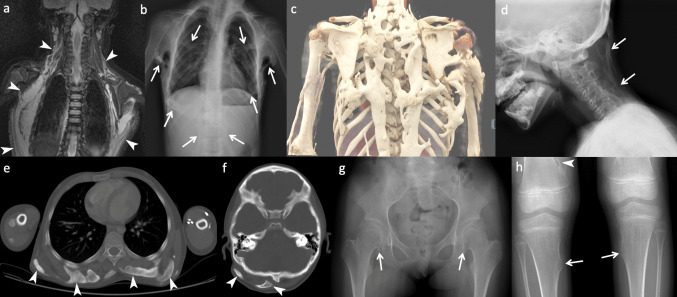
Imaging findings in fibrodysplasia ossificans progressiva (FOP). a Coronal short tau inversion recovery (STIR) image of a 13-month-old female (Patient 3) showing extensive myofascial edema (arrowheads) in the posterolateral thoracic wall at an early disease stage. b Follow-up radiography at age 6 years in the same patient demonstrates progression to heterotopic ossification of the thoracic wall, paraspinal, and axillary regions (arrows). c Three-dimensional volume-rendered image of an 8-year-old female (Patient 4) showing extensive, irregular ossifications involving the thoracic wall, paraspinal regions, and upper extremities. d Lateral cervical spine radiography of a 5-year-old male (Patient 8) demonstrating nuchal membrane ossification (arrows) and cervical facet joint ankylosis from C2 to C7, with enlarged posterior elements and hypoplastic vertebral bodies. e, f Axial CT images of the thorax (e) and head (f) from the same patient as in (d) showing thick subcutaneous, fascial, and/or intramuscular ossifications (arrowheads); the scalp lesion corresponds to a clinically described scalp nodule. g Anteroposterior (AP) pelvis radiography of the same patient as in a showing short, broad femoral necks with hypoplastic femoral heads, acetabular dysplasia, and small osteochondromas (arrows) at the medial femoral necks. h AP knee radiographs of another 6-year-old female (Patient 7) showing small sessile osteochondromas (arrows) of the proximal medial tibiae and a pedunculated osteochondroma (arrowhead) of the right distal femur
